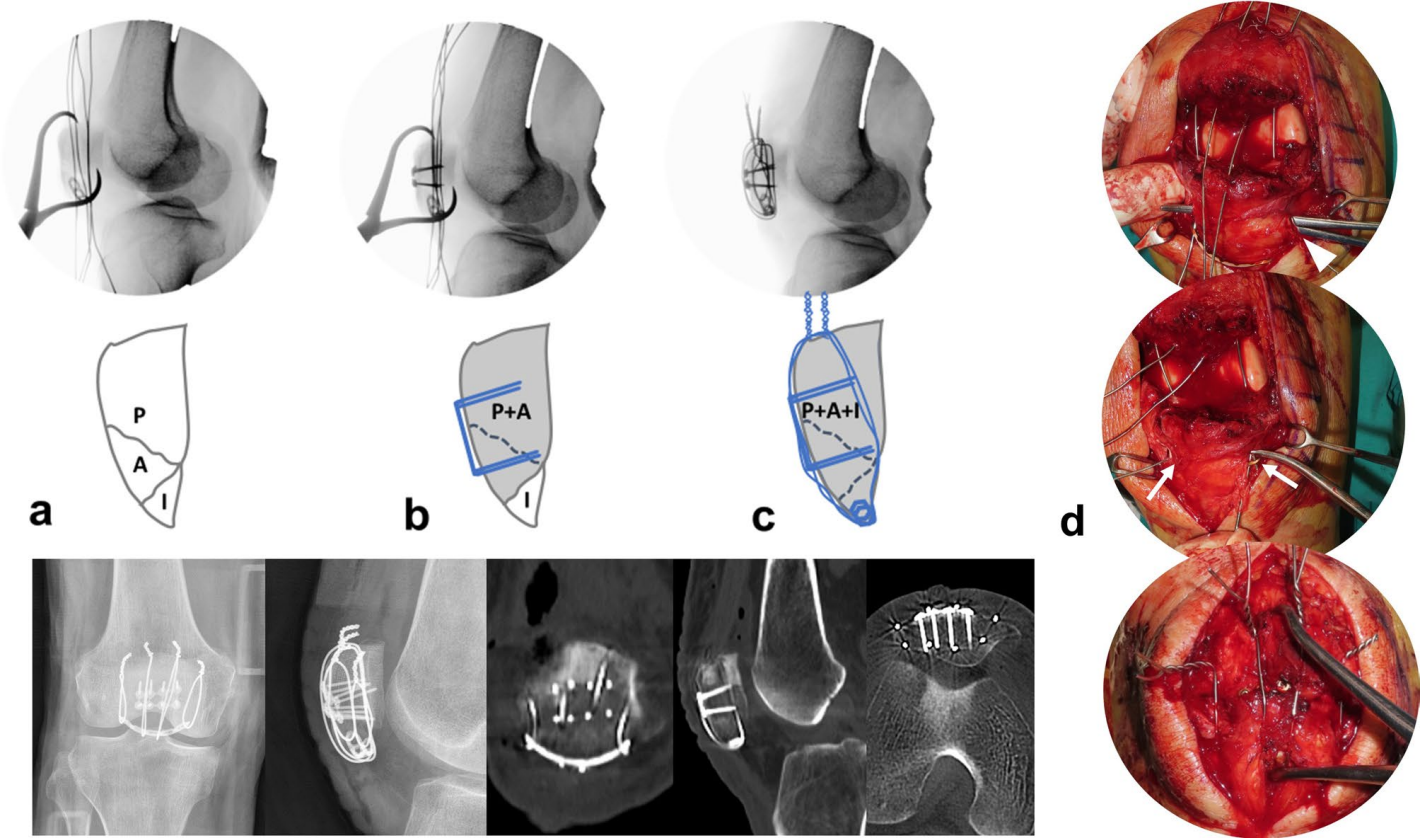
(a) A Proximal, Anterior, and Inferior pole fragment were reduced by reduction clamp with passed separate vertical wires engaged with a rim plate. (b) The Proximal and Anterior fragments were fixed by supplementary fixation using anterior cortical plate to be converted to type A from type C. (c) After conversion, rim plate augmented separated vertical wires were tightened. Postoperative radiographs show the rim-plate-augmented separate vertical wiring with supplementary fixation using anterior cortical plate. All identified fragments were anatomically stabilized. (d) The Mosquito was passed under the patellar tendon(white arrow head) before the rim plate passage. A medial and lateral wire(white arrow) went through both end holes of rim-plate. Middle wires were placed over the plate for wrapping.
